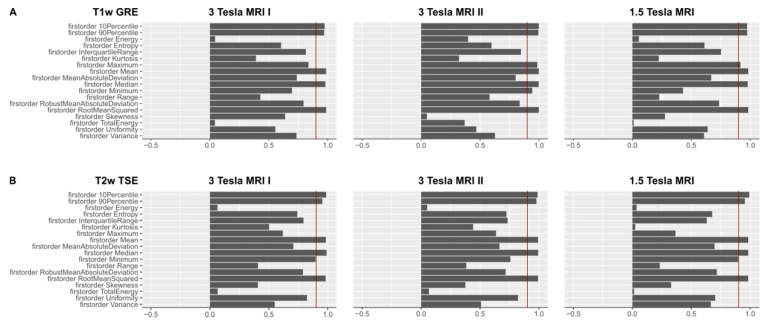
(A). OCCCs_20,30_ of the first-order features derived from T1w images are shown for the three different MR scanners. The red benchmark at 0.9 indicates excellent agreement in the OCCCs. Mean, median, RMS, 90th, and 10th percentile showed excellent agreement on all three MR scanners. On the 3 Tesla MR scanner II, also maximum and minimum achieved excellent agreement in the OCCCs. Mean, median, and RMS also showed excellent agreement in the OCCCs_10–30_ (not shown), whereas excellent agreement of the 90th and 10th percentile was inconsistent. A summary of the numerical results of the OCCCs on different scanners and pulse sequences is listed in Supplementary Material 5 (see Table SF5). Complete figures of OCCCs of all features, scanners, and sequences are shown in the Supplementary Files 6–8 (see Figures SF6–SF8). (B). OCCCs_20,30_ of the first-order features derived from T2w images are shown for the three different MR scanners. The red benchmark at 0.9 indicates excellent agreement in the OCCCs. Mean, median, RMS, 90th, and 10th percentile showed excellent agreement on all three MR scanners. On the 3 Tesla MRI I and the 1.5 Tesla scanner, also minimum achieved excellent agreement in the OCCCs_20,30_. Mean, median, and RMS also showed excellent agreement in the OCCCs_10–30_ (not shown), whereas excellent agreement of minimum, 90th and 10th percentile was inconsistent. A summary of the numerical results of the OCCCs on different scanners and pulse sequences is listed in Supplementary Material 5 (see Table SF5). Complete figures of OCCCs of all features, scanners, and sequences are shown in the Supplementary Files 6–8 (see Figures SF6–SF8).
